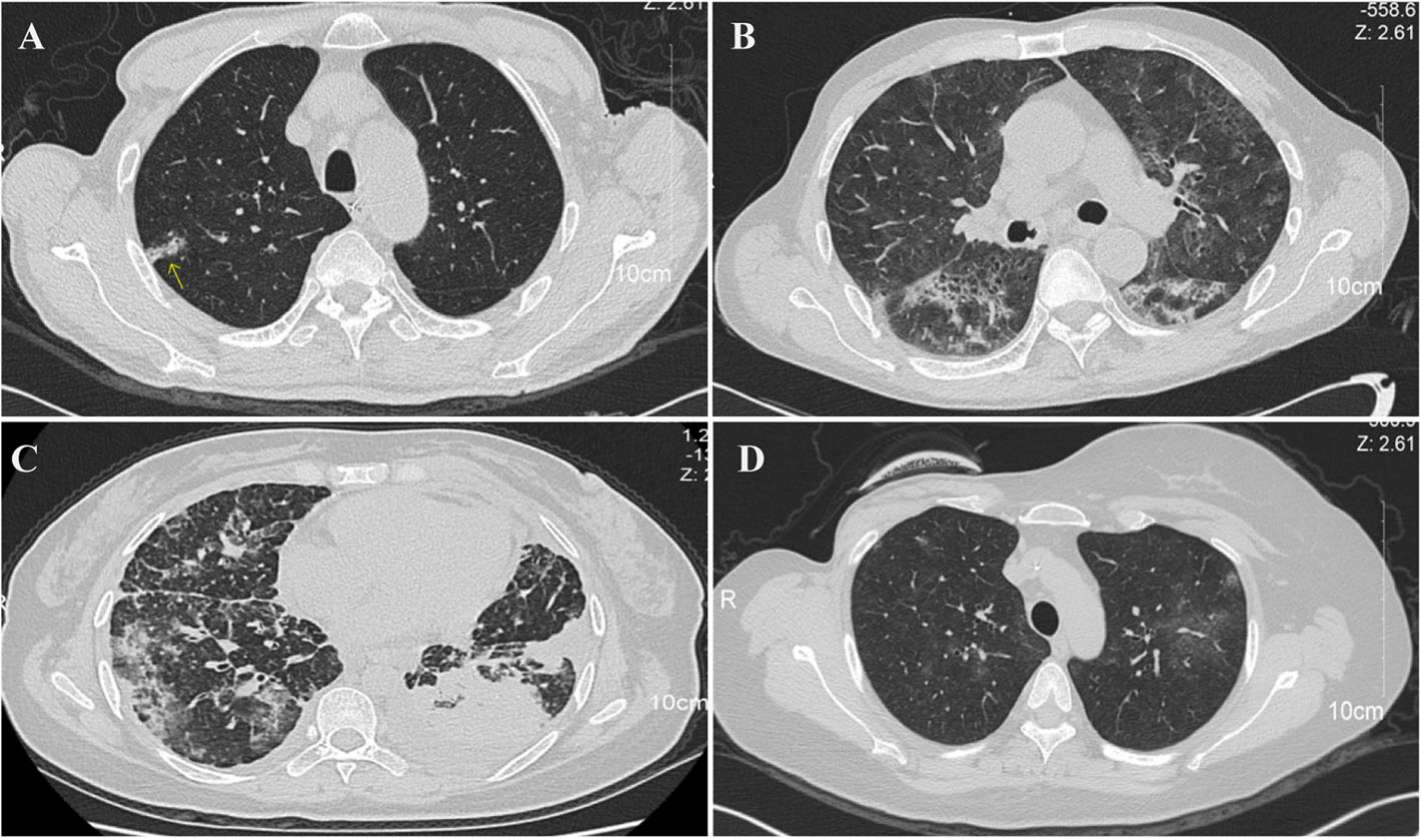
Chest CT images (transverse plane) of 4 clinical suspected COVID-19 patients. a Patient 1: right-sided multiple ground-glass opacities. b Patient 2: bilateral multiple ground-glass opacities. c Patient 3: bilateral multiple ground-glass opacities. d Patient 4: bilateral ground-glass opacities. Ground-glass opacities were indicated by an arrow in (A)
